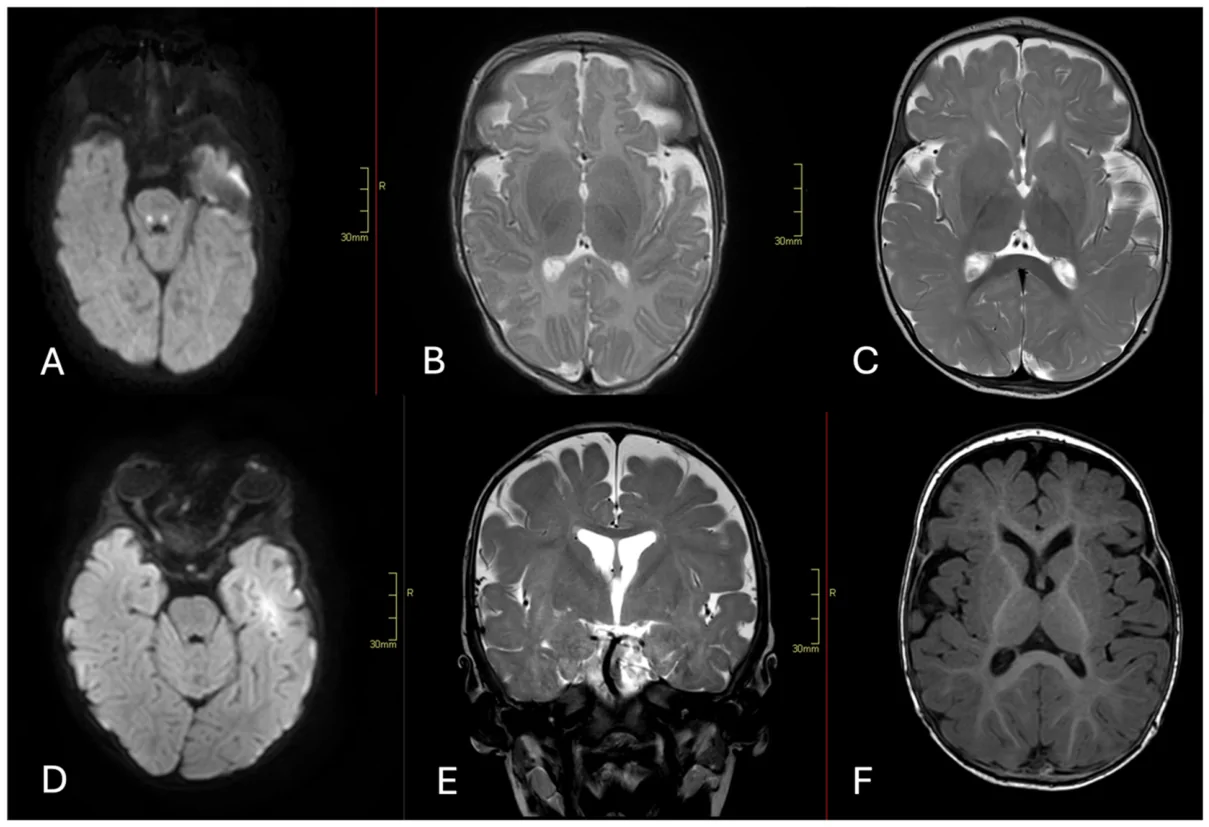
Brain MRI findings at initial presentation and after treatment. (A) (MRI study performed at three months of age) Diffusion-weighted imaging (DWI) showing restriction in the dorsal tracts of the brainstem. (B) (three months of age) and (C) (seven months of age): Axial T2-weighted imaging (T2WI) showing reduction in the size of the thalami and mild T2 hyperintense signal in the globi pallidi. (D) (study performed at six months of age): DWI showing resolution of the restriction in the dorsal tracts of the brainstem. (E) (six months of age): Coronal T2WI section showing mild T2 hyperintense signal in the globi pallidi. (F) (six months of age): Axial T1-weighted imaging (T1WI).
